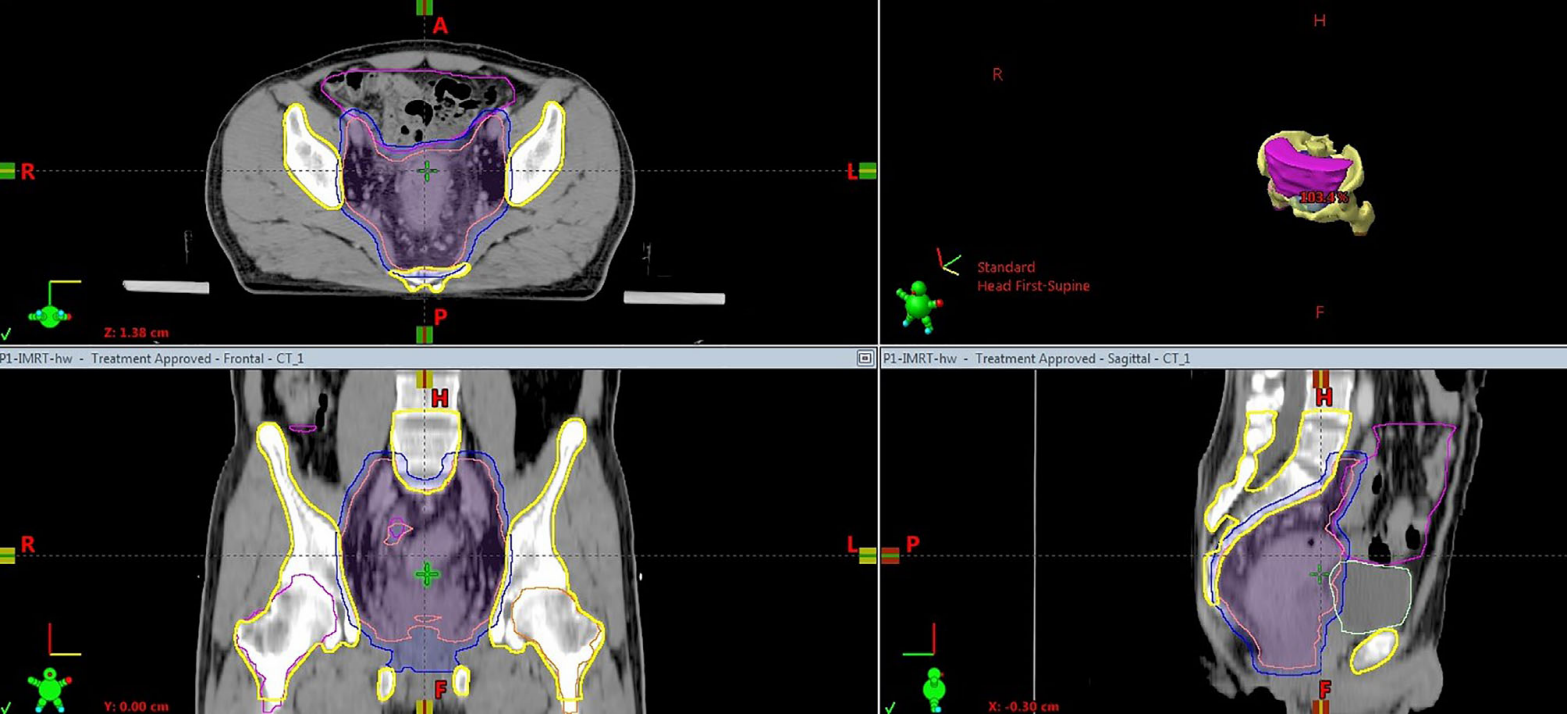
Computed tomography slice showing the delineation of target volume and OARs. Pink solid, clinical target volume; blue solid, planning target volume; yellow, pelvic bone marrow; magenta, small bowel; light green, bladder.
